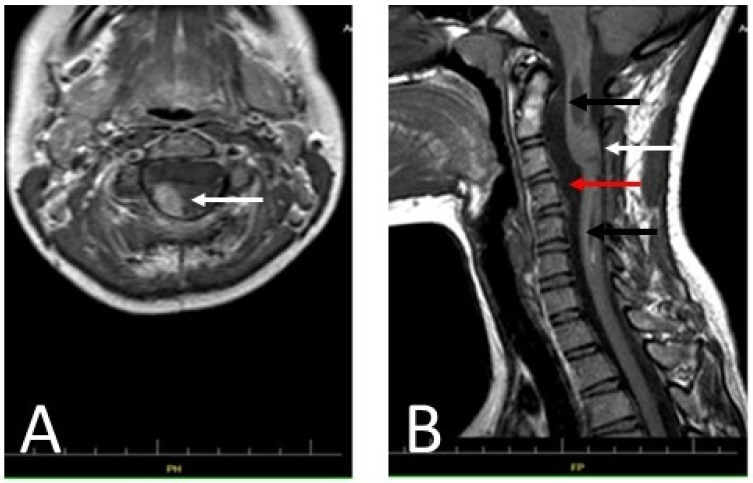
Diagnostic T1-weighted MRI images of the patient’s cervical spine: (A) An axial section at the C2 level of the patient’s cervical spine made after administration of a contrast agent. The remainder of the tumor showed no apparent progression compared to the MRI performed 48 h after the first treatment (arrow). (B) A sagittal section of the patient’s cervical spine (without contrast). The photo shows decompression of the syringomyelic cavity (black arrows) and the remains of the partially removed tumor, visible as an isointense lesion in the spine (white arrow). The reserve of cerebrospinal fluid in the spinal canal is clearly increased (red arrow). The photo shows no signs of tumor progression.
